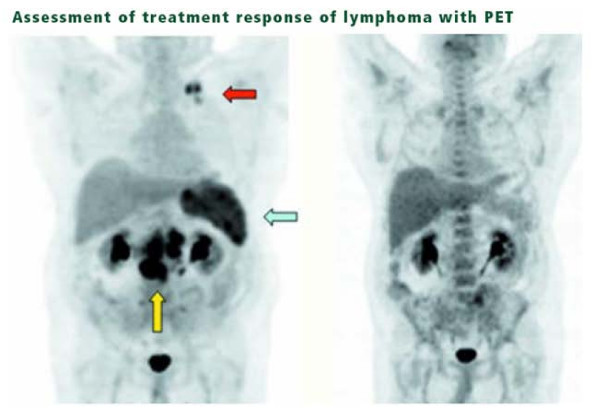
Assessment of treatment response of lymphoma with PET. Images of pre- and post-therapy PET scans in a lymphoma patient treated with chemotherapy. The pre therapy image (left) shows increased FDG uptake in the left supraclavicular region (red arrow), mesentery, retroperitoneum (yellow arrow), and spleen (olive arrow). The post-therapy image (right) shows no residual disease, with a bone marrow activation commonly seen after chemotherapy and which can be seen with other treatments such as granulocyte colony-stimulating factor.
